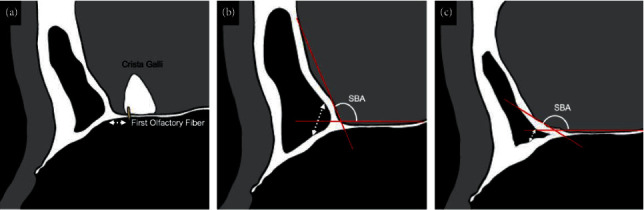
Relationship between the floor of the frontal sinus, the posterior wall of the frontal sinus, and the first olfactory fibre in sagittal computed tomography. (a) Relationship between the first olfactory fibre and the posterior wall of the frontal sinus. (b, c) Relationship between the skull base-frontal sinus angle (SBA) and the posterior wall of the frontal sinus.
